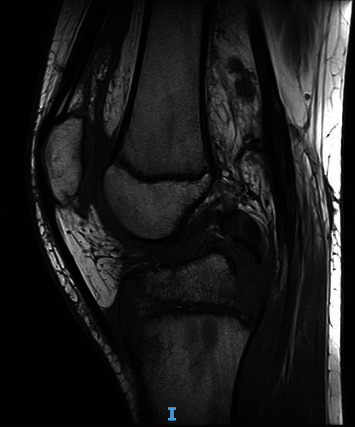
MRI of Case 1. The sagittal view shows PCL avulsion from the posterior tibial plateau. Growth cartilages are visible on both femur and tibia.
